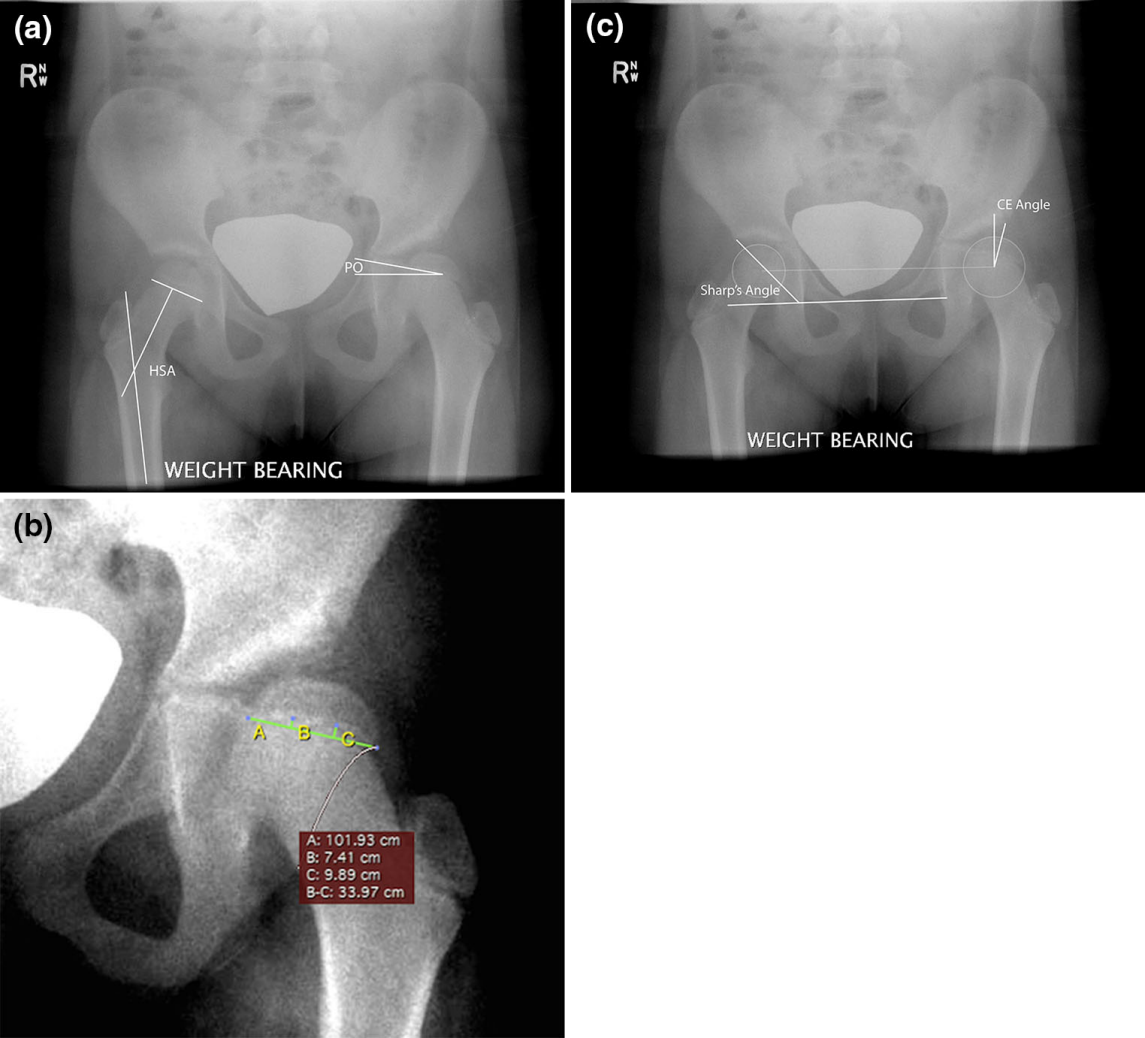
Radiographic measurements: a the head–shaft angle (HSA) is the angle between a line perpendicular to the proximal femoral physis and the femoral shaft. The physeal orientation (PO) is the angle between the horizon and the line through the middle 1/3 of the proximal femoral physis. b The middle 1/3 of the proximal femoral physis was defined by a line connecting the medial point (*point B*) and lateral point (*point C*) of the middle 1/3 of the physis. c Sharp’s angle is measured from the radiographic teardrop to the lateral margin of the acetabulum and a line connecting both teardrops. The center edge angle of Wiberg (CE) is measured from the center of the femoral head to the lateral margin of the acetabulum and a line perpendicular to a line connecting the center of both femoral heads
